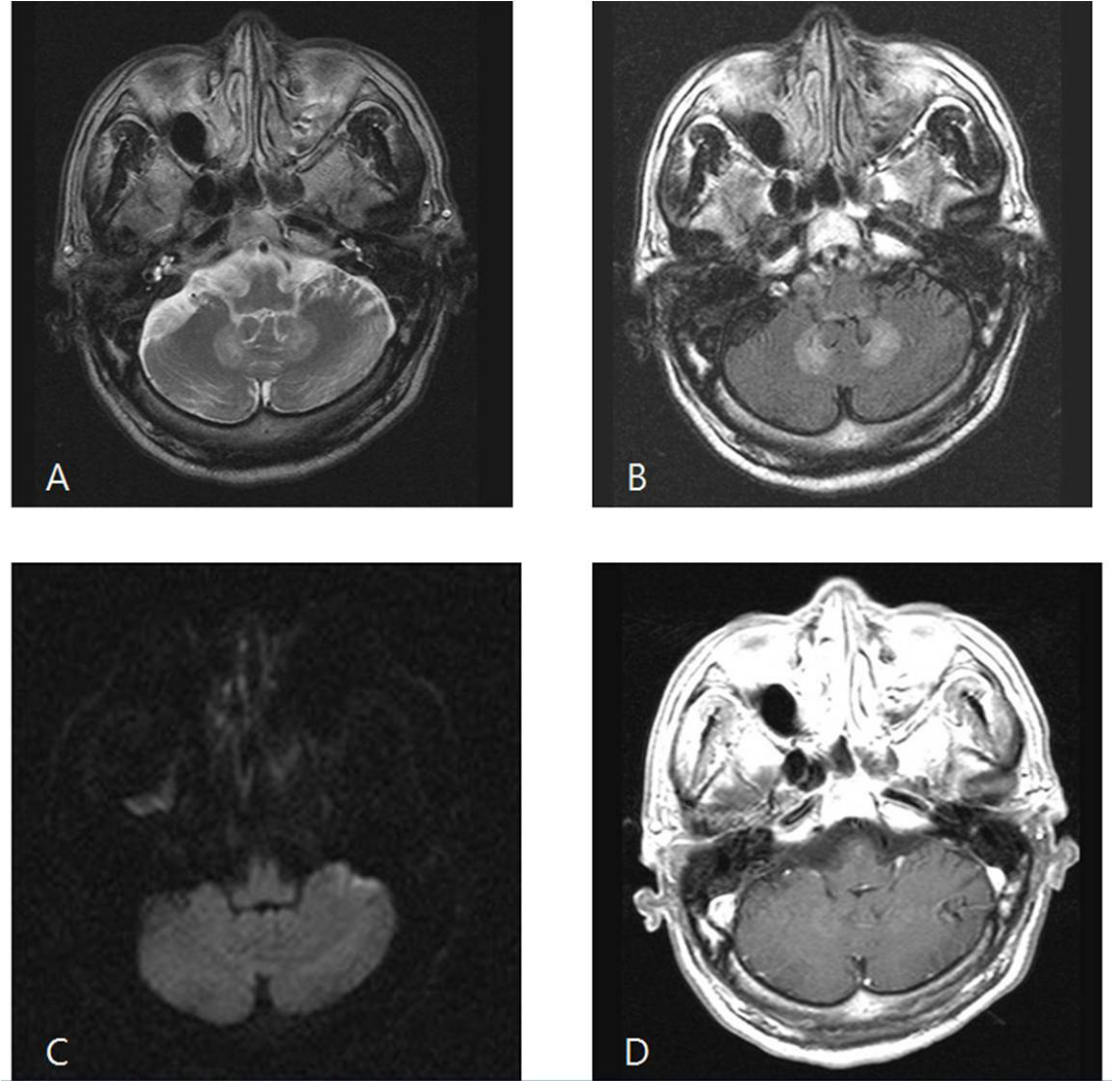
Initial MRI findings. A: T2-weighted image shows symmetrically increased signal intensities in the dentate nucleus of the cerebellum. B: FLAIR image shows symmetrically increased signal intensities in the dentate nucleus of the cerebellum. C: Diffusion weighted image shows no abnormality. D: Postgadolinium T1-weighted image shows no abnormality.
